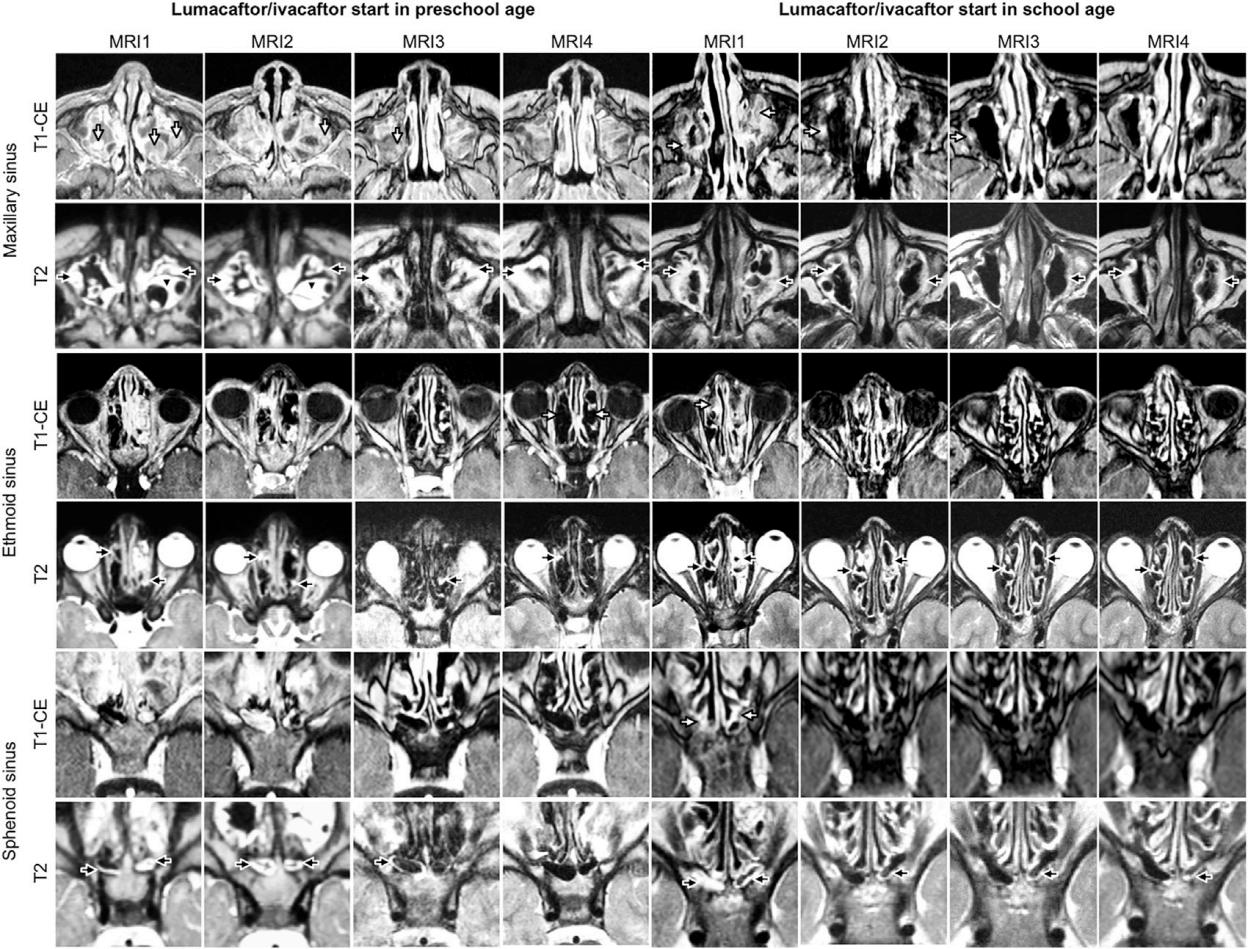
Representative examples of longitudinal magnetic resonance imaging of chronic rhinosinusitisparansal sinus abnormalities before (MRI1) and under therapy with lumacaftor/ivacaftor (MRI2-4) in children with cystic fibrosis starting lumacaftor/ivacaftor therapy in preschool and school age. Mucosal swelling is indicated by black arrows, mucopyoceles by white arrows and polyps by black arrowheads. Note the reduction of mucopyoceles in the maxillary sinus in the patient starting therapy in school age. The chronic rhinosinusitis magnetic resonance imaging (CRS-MRI) score in the patient starting therapy in preschool age (4 years at MRI1) was in MRI1 33, in MRI2 33, in MRI3 30 and in MRI4 29 and in the patient starting therapy in school age (9 years at MRI1) in MRI1 32, in MRI2 30, in MRI3 27 and in MRI4 30.
